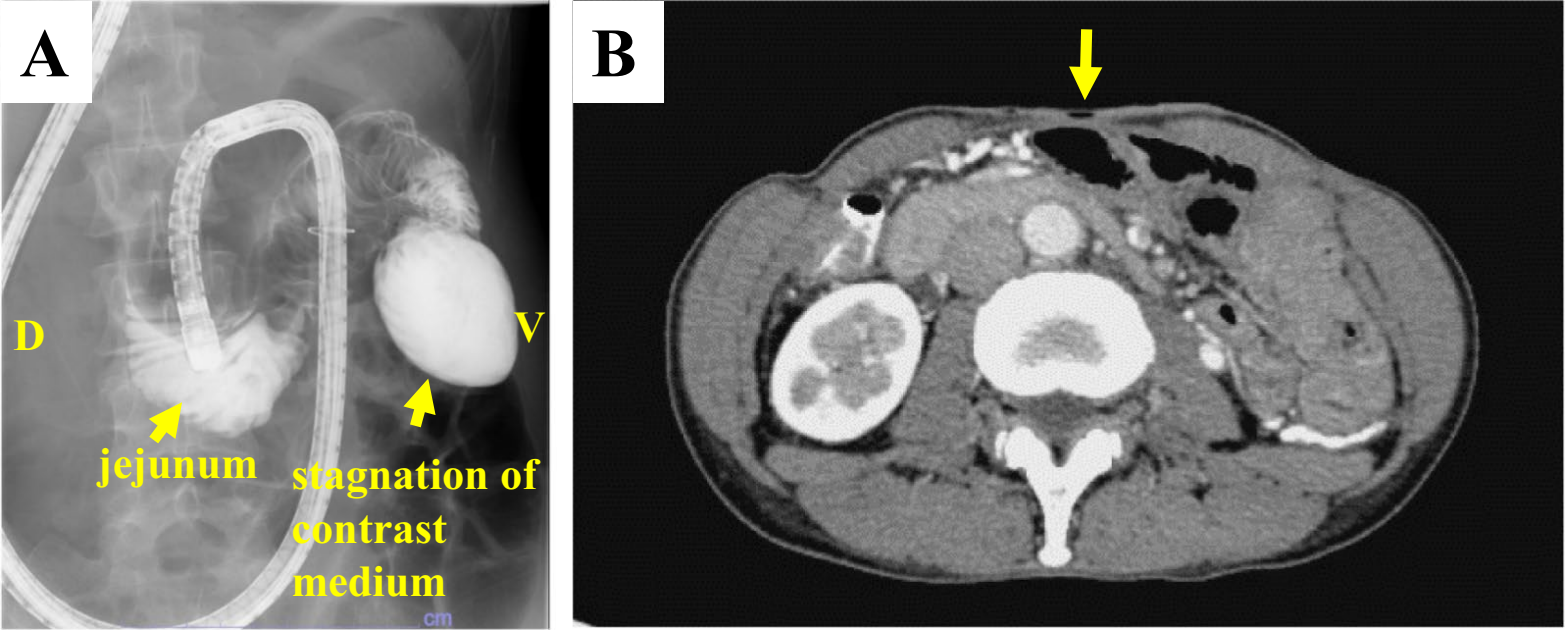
Preoperative imaging examinations are depicted. A Enteroclysis revealed stagnation of contrast medium in the jejunum near the ligament of Treitz due to strong flexion. (D: dorsal position V: vertical position). B Computed tomography revealing a mass in the dilated bowel, inferior to the fistula. The yellow arrows indicate the presence of an enterocutaneous fistula
